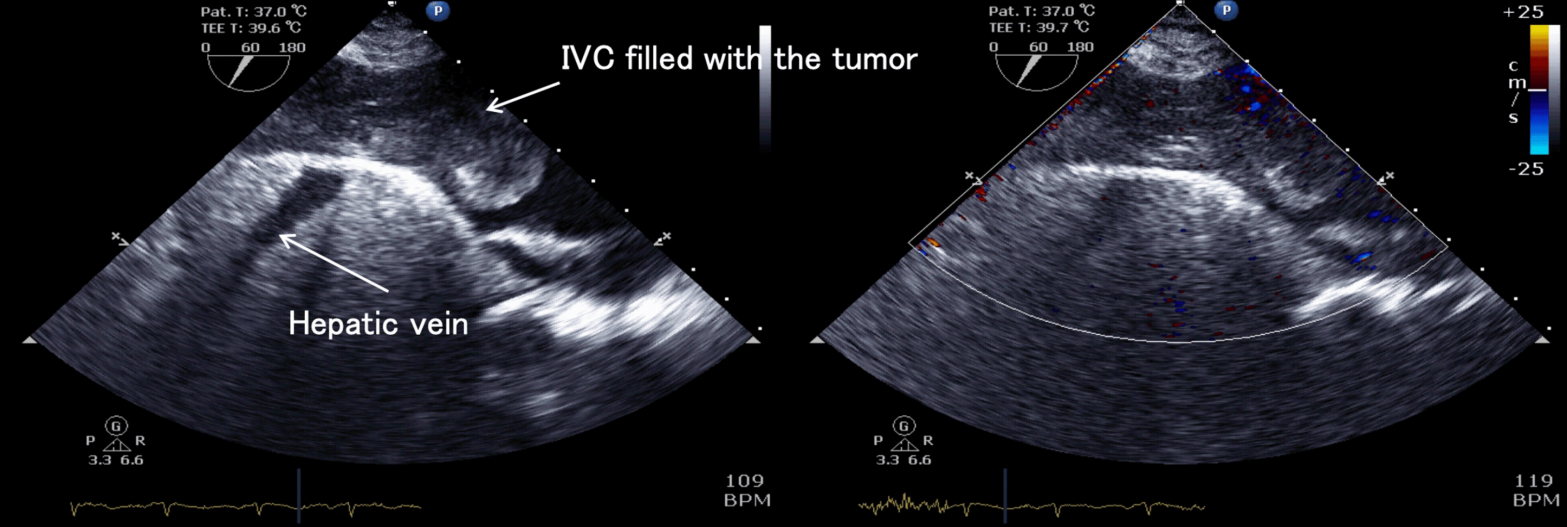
Intraoperative transesophageal echocardiography, transgastric inferior vena cava/hepatic veins view with color Doppler mode The inferior vena cava was filled with tumors, and blood flow in the inferior vena cava could barely be detected even when lowering the velocity scale. The tumor appeared to be fixed to the inferior vena cava wall without movement. The leading edge of the tumor extended to the right atrium, and the portion of the tumor within the right atrium showed to-and-fro mobility in synchrony with the cardiac cycle. No tumor was observed within the hepatic veins. IVC, inferior vena cava
